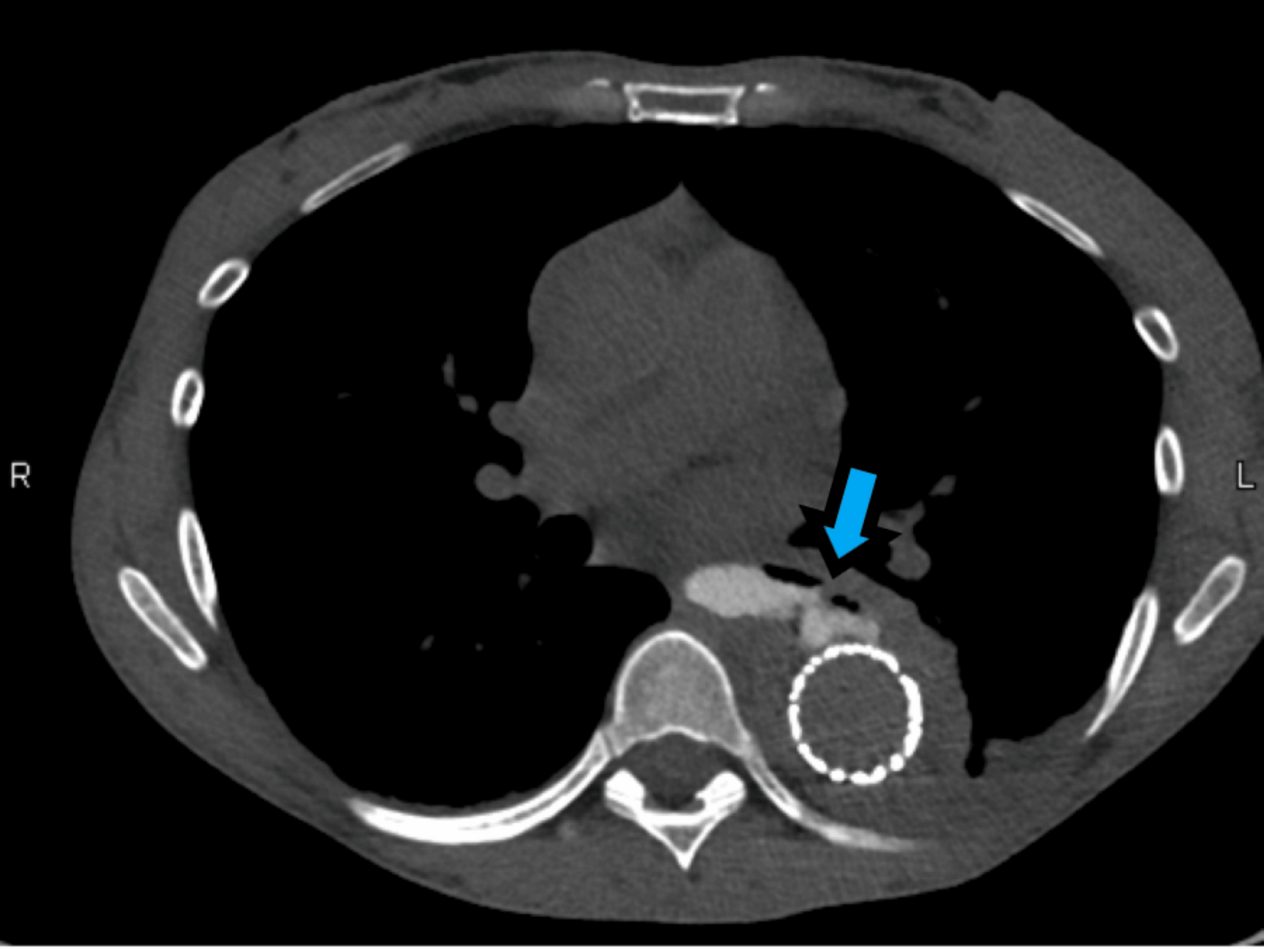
Computed tomography esophagram, axial plane, demonstrating esophageal perforation communicating with the false lumen of the thoracic aorta status post thoracic endovascular aortic repair.
